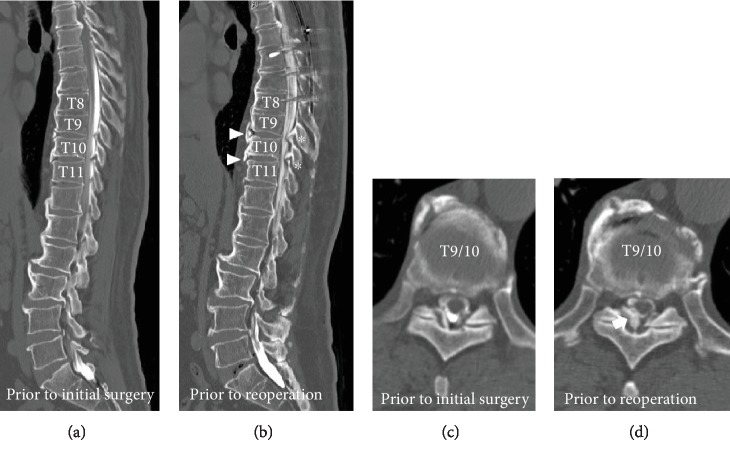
CT myelography images prior to initial surgery and reoperation. (a) Sagittal image prior to initial surgery. (b) Sagittal image prior to reoperation. Osteophytes (arrowheads) and markedly enlarged ossification of the yellow ligament lesion (asterisks) were observed at T9-11. (c) Horizontal image of T9-10 prior to initial surgery. (d) Horizontal image of T9-10 prior to reoperation. The ossification of the yellow ligament lesion is markedly enlarged (arrow), and spinal cord compression is apparent, which has shifted significantly to the left.
